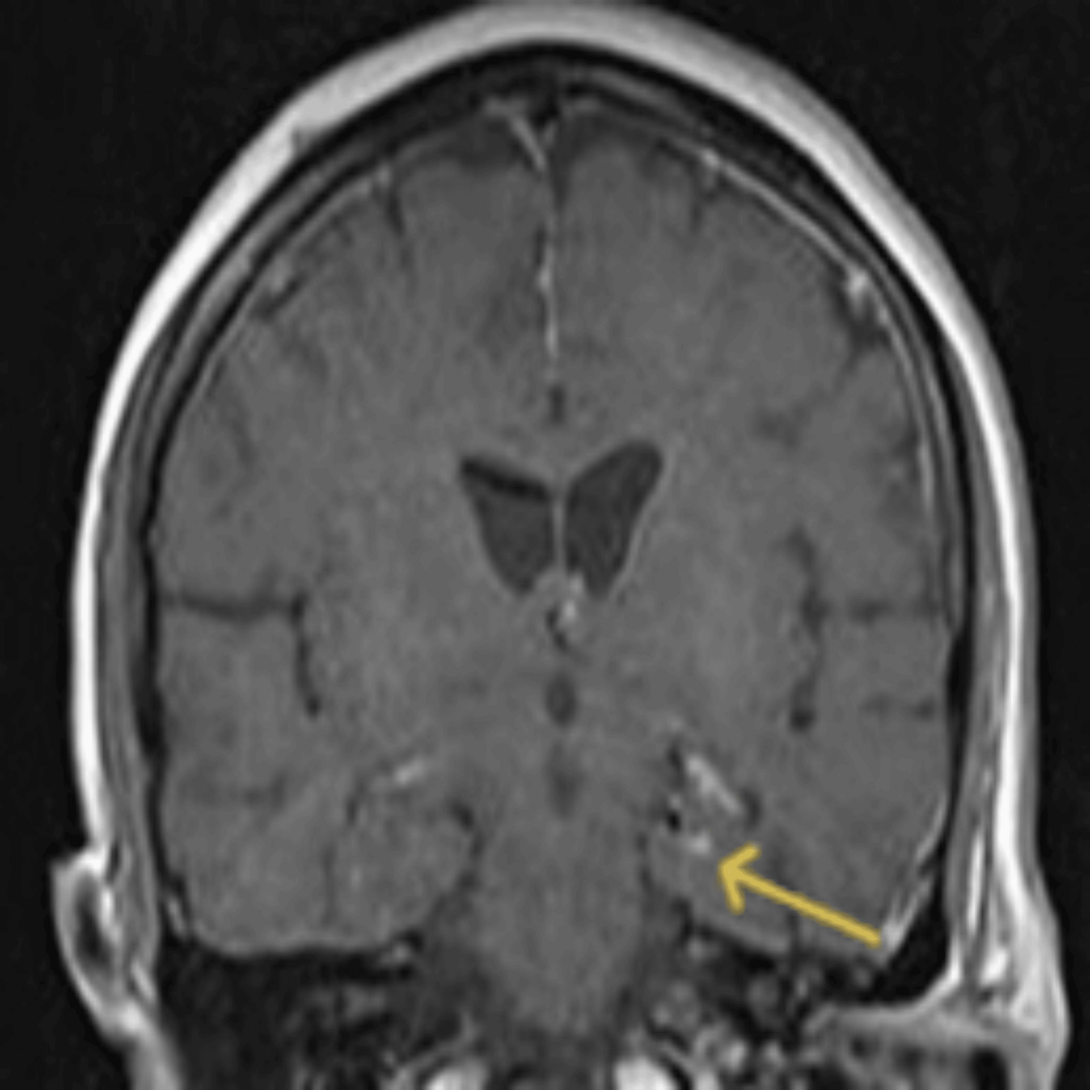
Coronal gadolinium-enhanced T1-weighted MRI The arrow reveals a spot-like enhanced lesion within the left temporal lobe area on the edge of the body of the left hippocampus of the brain.
